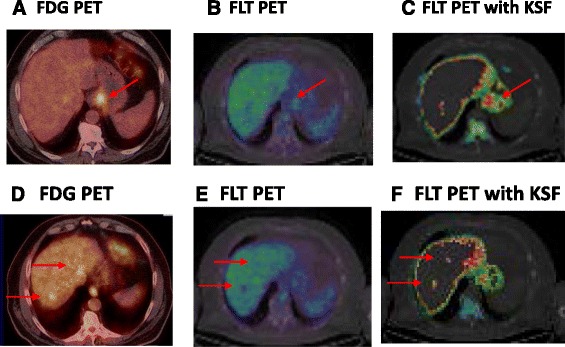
Transverse sections from a patient with gastroesophageal junction tumour with liver metastasis. On [^18^F]FDG-PET imaging, both the primary (a) and liver lesions (d) are visible. Whilst the primary oesophageal lesion is visible on the unfiltered [^18^F]FLT-PET imaging (b), visualisation is improved following application of the KSF (c). Liver metastases appear as an area of relative photopaenia (e) on [^18^F]FLT-PET imaging. Following application of the KSF, there is marked reduction of the background hepatic signal allowing for improved visualisation of hepatic metastases (f). Both lesions are also seen on baseline [^18^F]FDG-PET imaging (d)
